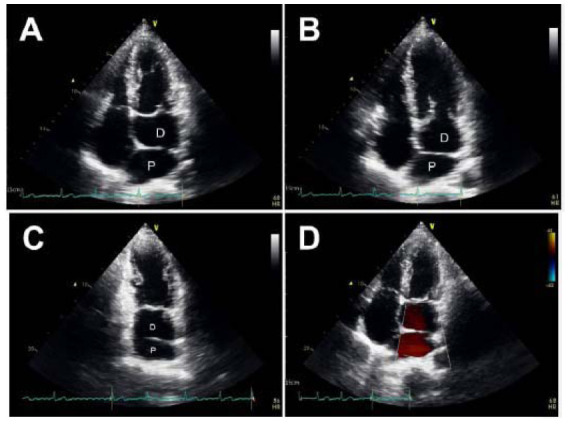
(A, B) Transthoracic cardiac sonography revealed an accessory septum at the left atrium, which divided the left atrium into a proximal chamber (P) and a distal chamber (D). (C) There was an obvious opening between the proximal chamber and the distal chamber. (D) Doppler echocardiography revealed no obvious turbulent flow in the left atrium.
